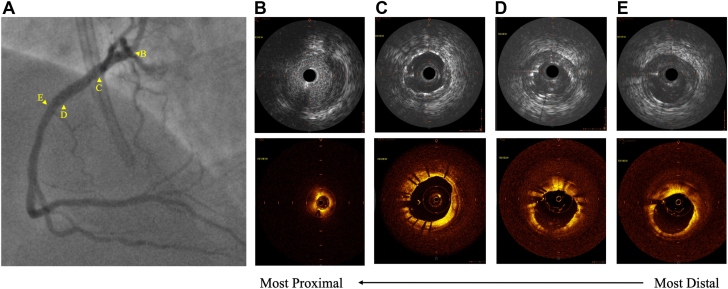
After percutaneous coronary intervention. (A) Angiogram of right coronary artery (RCA) lesion; intravenous ultrasound (top) and optical coherence tomography (bottom) visualizations of (B) proximal edge of stent struts of ostial RCA (demonstrating no extension into the aorta), (C) minimal stent area of 9.40 mm^2^, (D) distal stent edge struts and wire artifact, and (E) the distal reference.
